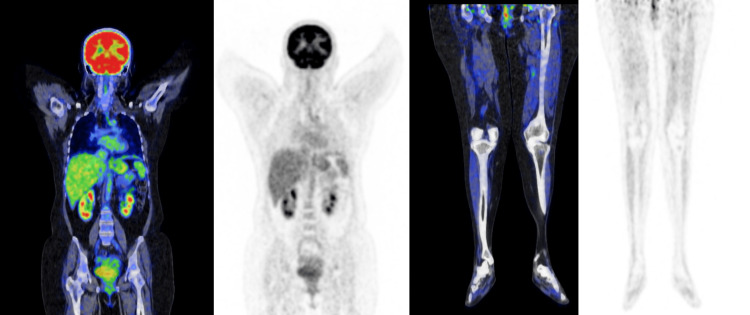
A whole-body FDG-18 PET/CT scan was negative for any other possible site of melanoma. FDG-18 PET/CT: ^18^fluorodeoxyglucose (FDG) positron emission tomography/computerized tomography
